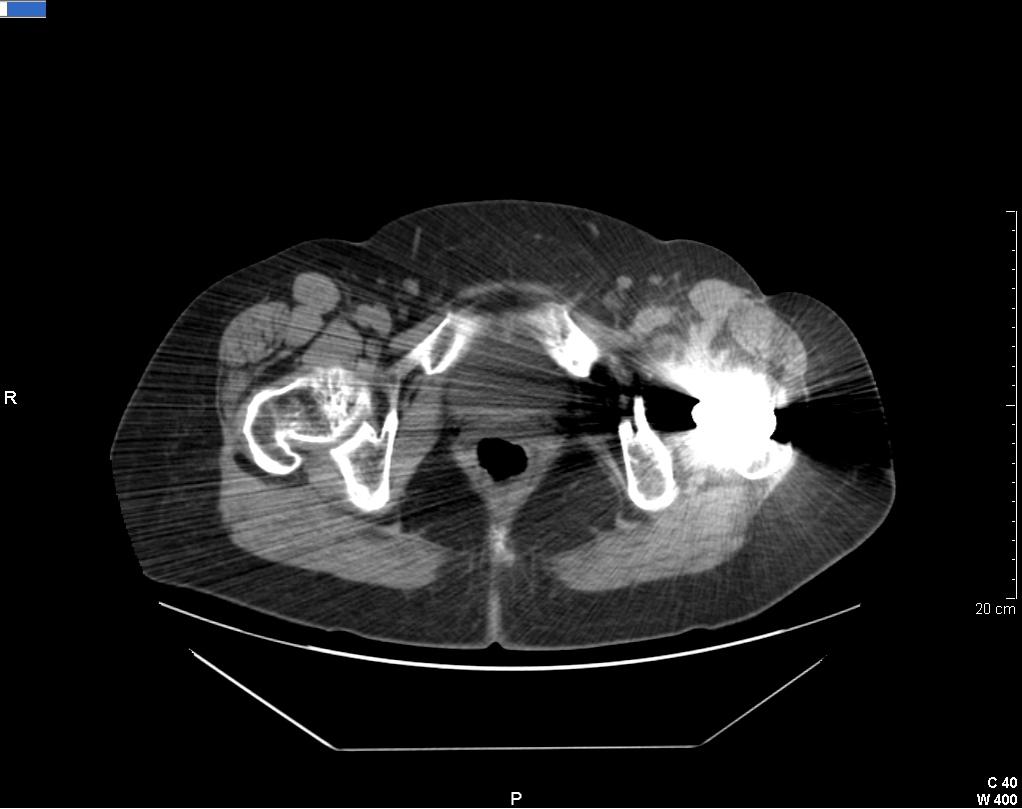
An image of a computed tomography scan performed 12 months after treatment. Regression of the iliopsoas abscess is shown. The cutaneous fistula is completely healed.
